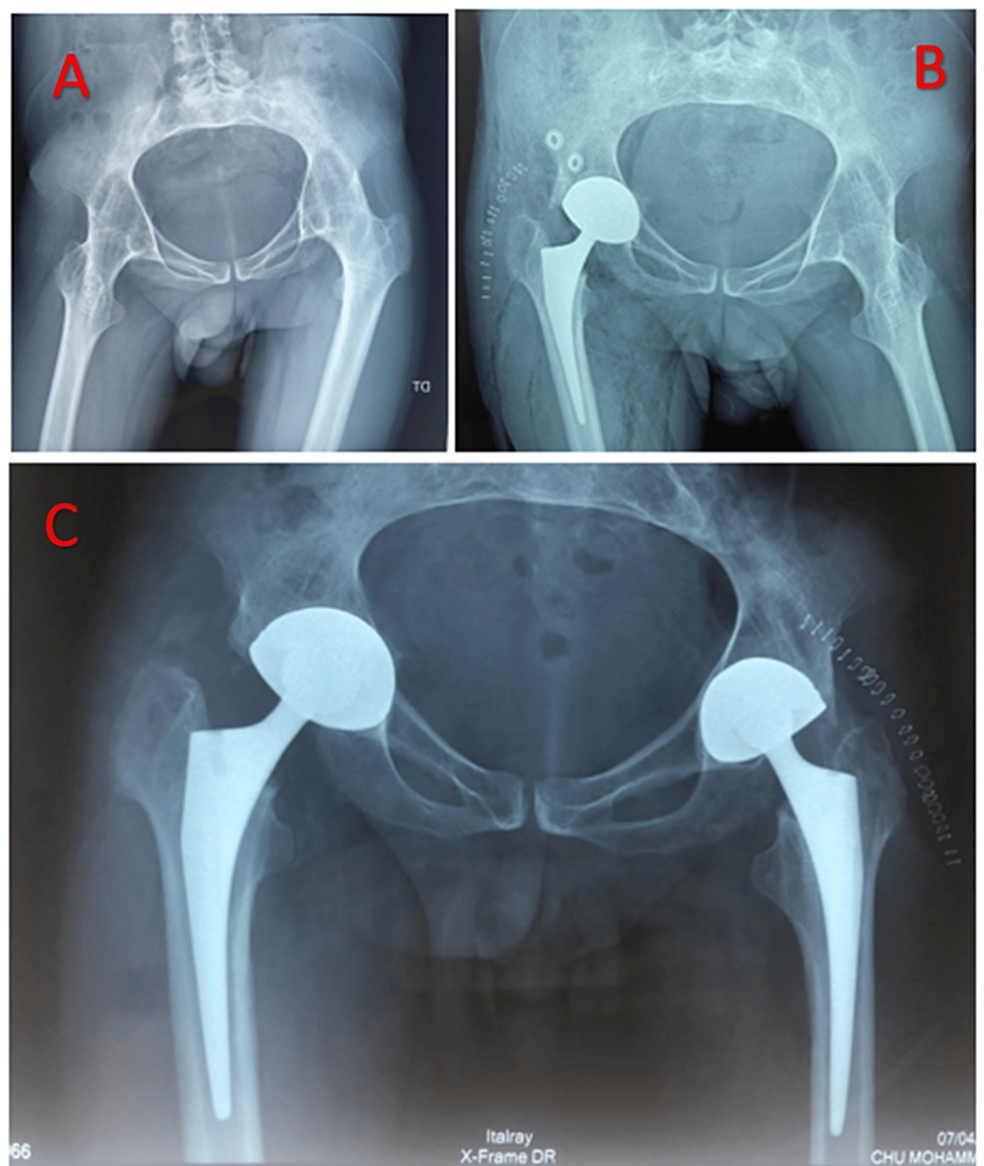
Radiographic findings in the case 3 patient with an abnormal location of the sciatic nerve A: Front pelvis X-ray showing bilateral ankylosis of both hips secondary to ankylosing spondylitis. B: Postoperative control pelvis radiograph after the first prosthesis on the right, which passed without complication. C: Postoperative control pelvis radiograph after the second prosthesis on the left, during which this anatomical peculiarity was revealed.
